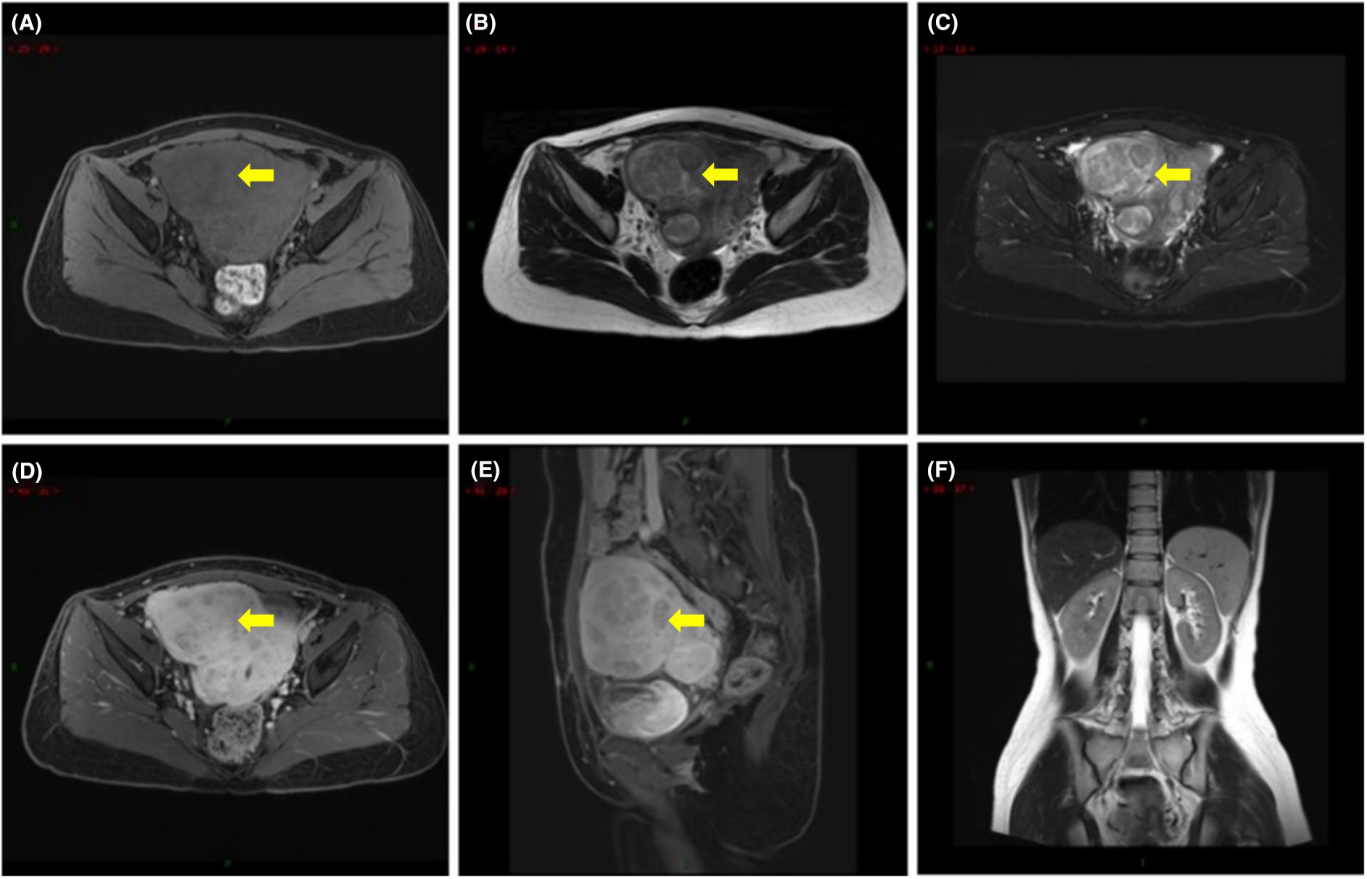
Magnetic resonance imaging (MRI) before hysterectomy. (A) Axial fat‐saturated precontrast T1‐weighted image showing intermediate signal intensity foci. (B) Axial T2‐weighted image showing intermediate and hypointense foci. (C) Axial fat‐saturated T2‐weighted image showing intermediate and hypointense foci. (D) Axial fat‐saturated postcontrast T1‐weighted image showing inhomogenous enhancement of the foci and lower signal intensity of the foci compared to the myometrium. (E) Sagittal fat‐saturated postcontrast T1‐weighted image showing inhomogenous enhancement of the foci and lower signal intensity of the foci compared to the myometrium. (F) Coronal T2‐weighted image showing normal kidney.
